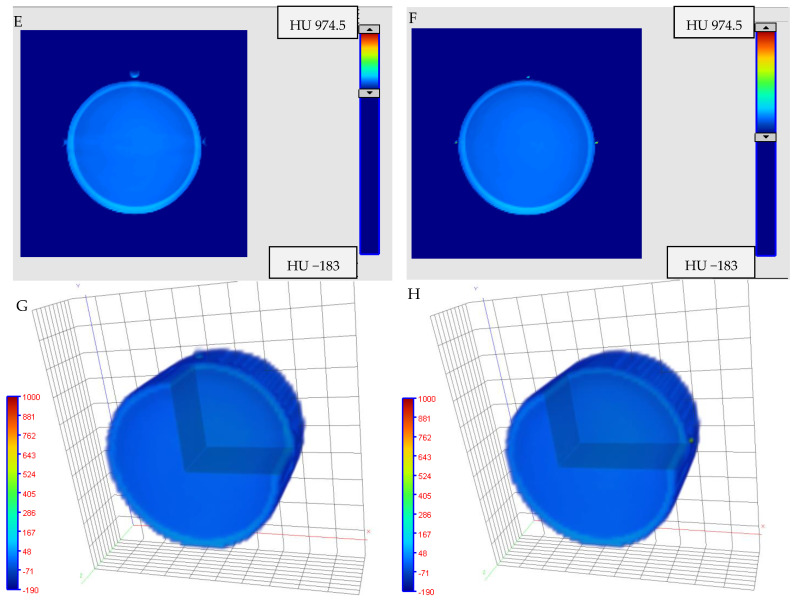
Two-dimensional (transversal plane, Z = −1 mm) and three-dimensional images of PH-6-DD2 container (GeVero Co.) with 5% gelatine (iCBCT, Pelvis, TrueBeam accelerator, Varian). The following markers were attached to the container at 0, 90 and 270°: steel wire commonly used as a marker in CT (1 mm thickness) (A,C), reflective marker spheres (Brainlab) (B,D), vitamin (E) of the shape of round capsules (E,G) and the shielded copper wire of the network cable (0.5 mm thickness) (F,H). The green circle in (A) indicates a cylindrical VOI of 32.8 mm in diameter and 2 mm long, applied to each scanned container with different markers. The red line in (B) is the position of the profiles applied to each scanned sample (see Figure 5). iCBCT images were processed with the polyGeVero-CT and polyGeVero (GeVero Co.) software packages.
